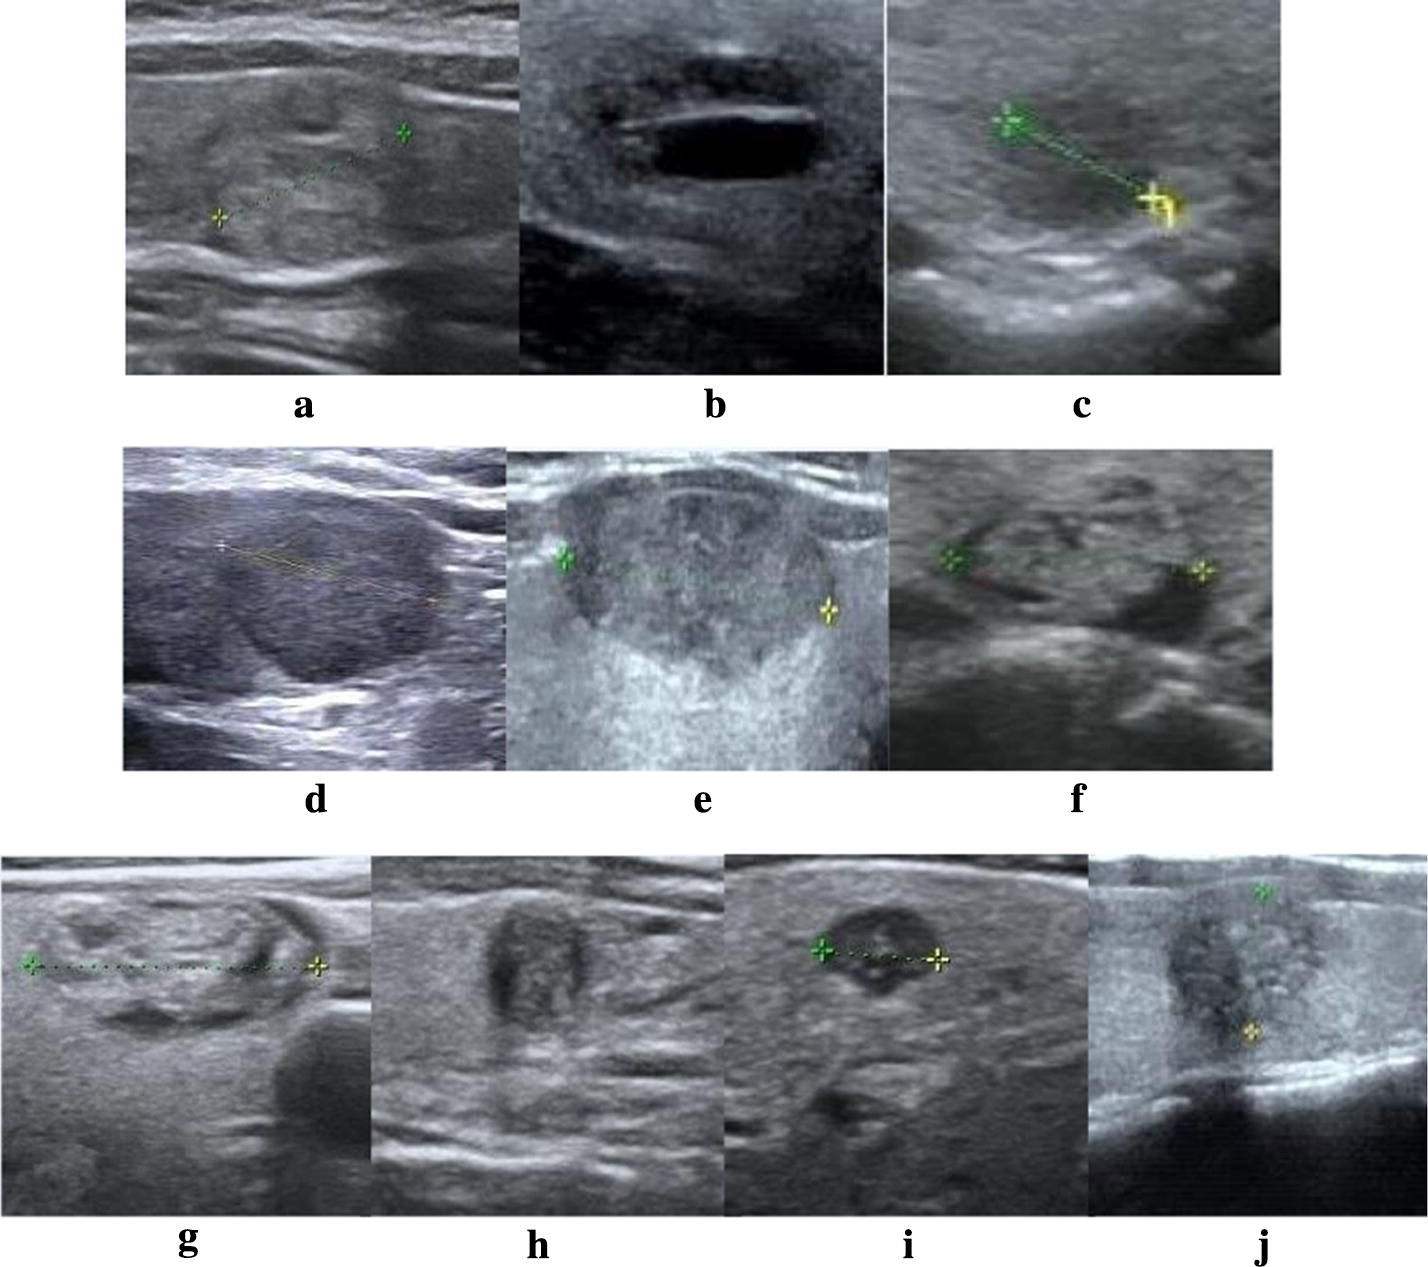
Samples of correct classification results for Ultrasound images of thyroid nodules. Each image is labeled with the TIRADS classification in Table 6
